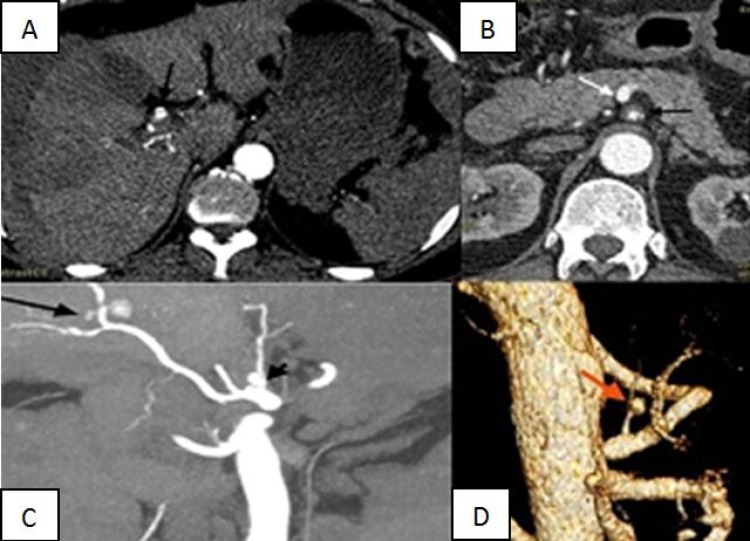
A) Axial contrast-enhanced CT (CECT) scan demonstrate contrast filled saccular out-pouching at the level of the left hepatic artery (black arrow). Hemoperitoneum with areas of higher attenuation indicates acute active bleeding is present. Peripheral segmental liver hypo-densities due to de-vascularization and vasospasm are also evident. B) Axial CECT through the celiac trunk level shows left gastric artery aneurysm with intraluminal thrombosis. C) Coronal Maximum Intensity Projection (MIP) reformation demonstrates concomitant aneurysm of hepatic artery (long arrow) and left gastric artery (short arrow). D) A volume-rendered 3D CT angiography image displays a small saccular left gastric artery aneurysm
